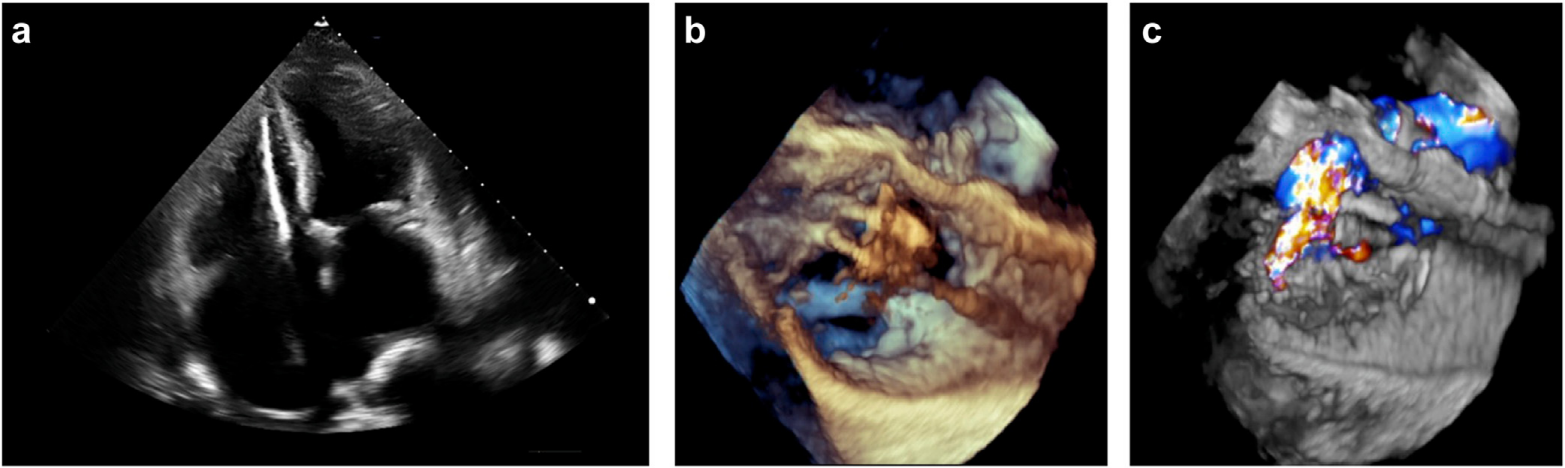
Echocardiography demonstrating device-related severe tricuspid regurgitation. (a) Apical 4 chamber view showing the ventricular lead adjacent to the septal leaflet of the tricuspid valve. (b) Three-dimensional echocardiographic view constructed from transesophageal images showing a surgeon’s view of the tricuspid valve with the device lead impinging on the septal leaflet with (c) resultant severe tricuspid regurgitation.
